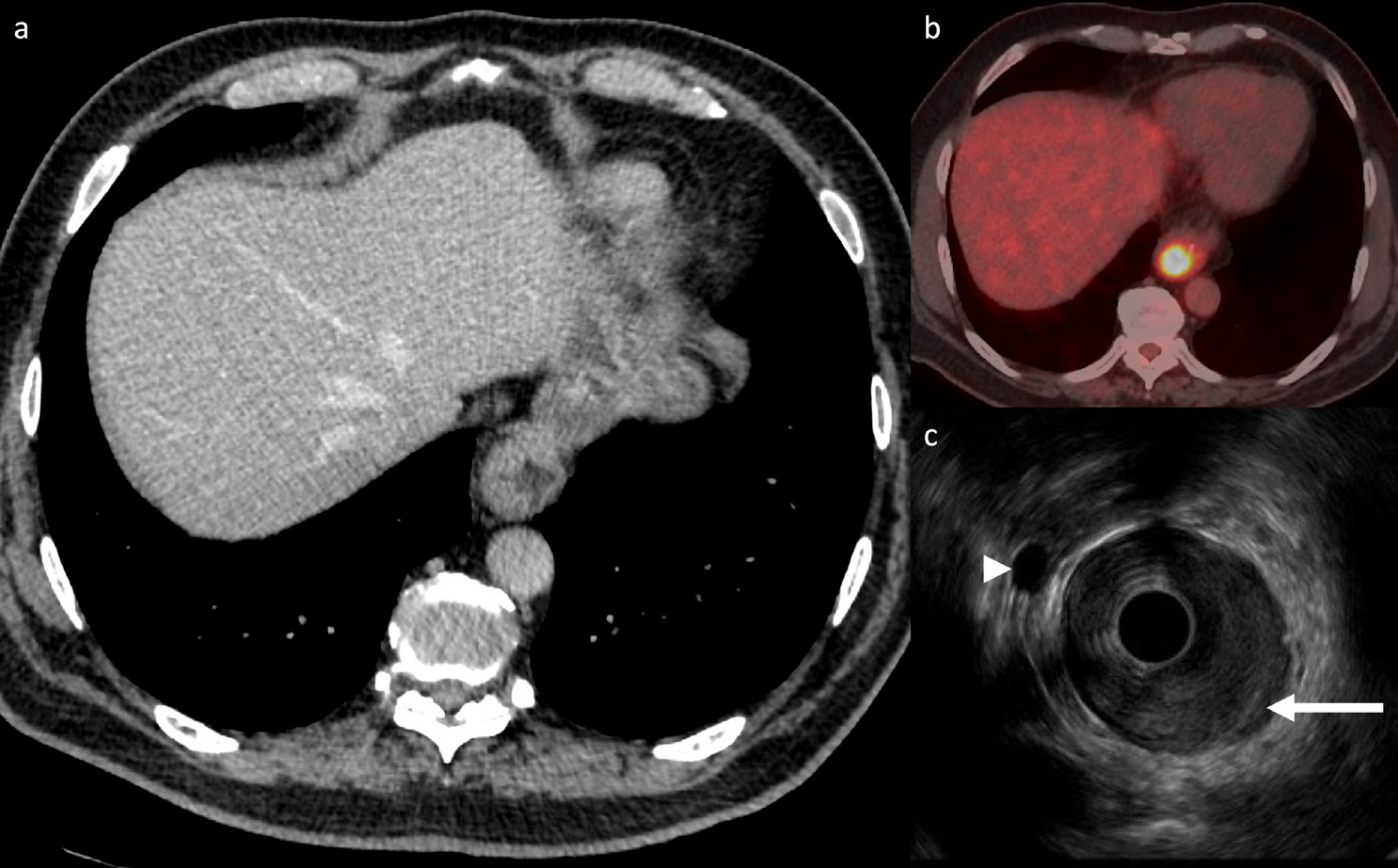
Axial CT showing mural thickening of the distal oesophagus (a), axial
